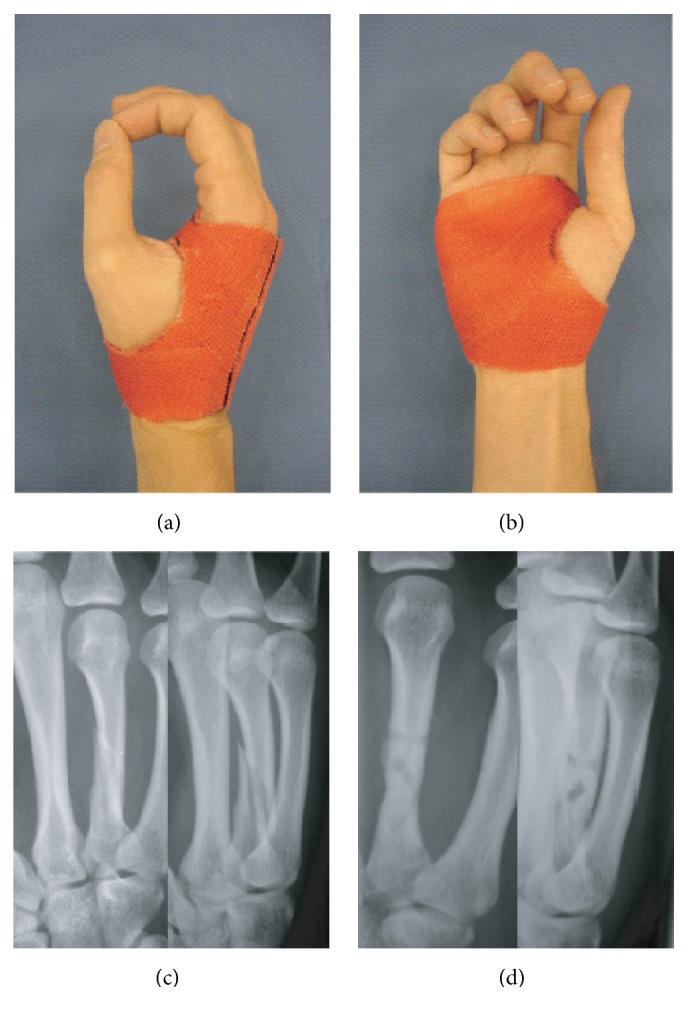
Middlehand orthoses (fingers excluded): (a) dorsoradial and (b) palmar view; case sample: displaced 4th metacarpal fracture, (c) X-ray on admission and (d) at 6 weeks following open reduction and fixation (resorbable implant; 2 mm LactoSorb® plate) (DASH analysis of case series: *n* = 15; score-mean = 32.07 ± 3.54 SD; DASH follow-up interval (weeks): 11.57 ± 0.77 SD).
